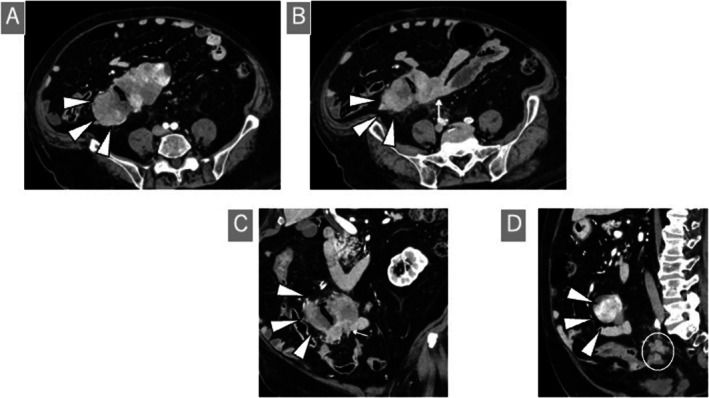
Preoperative contrast‐enhanced computed tomography (CT). Abdominal contrast‐enhanced CT tomography showing a 13 cm tumor in the right lower abdomen. (A–C) Air density within the tumor suggests intestinal penetration with abscess formation. The lesion extended from the terminal ileum to the mesentery and retroperitoneum, displacing the adjacent small intestine. (B, C) Suspected disseminated nodules are visible anterior to the sacrum. (D) Axial view showing the relationship between the tumor and the surrounding structures.
